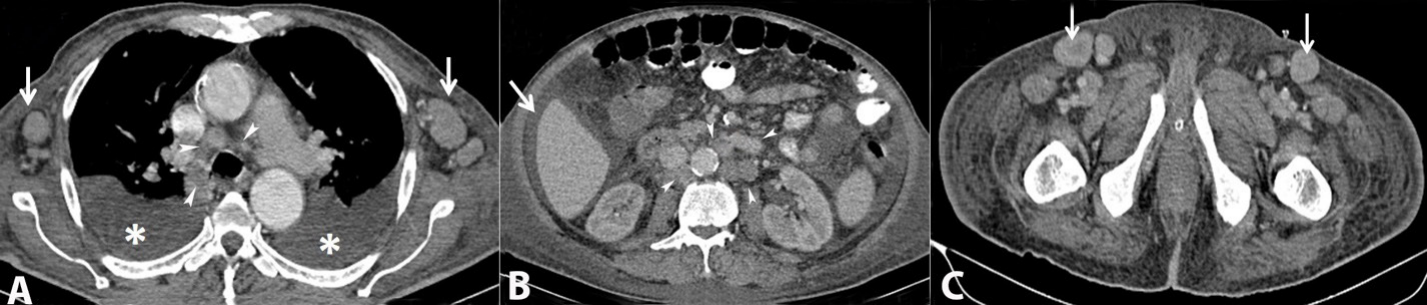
A panel of contrast-enhanced axial CT scan images in soft-tissue window showing: A – Thoracic CT - bilateral pleural effusions (asterisk), bilateral axillary lymphadenopathy (arrows) and mediastinal lymphadenopathy (arrowheads); B – Abdominal CT - ascites (arrow) and retroperitoneal lymphadenopathy (arrowheads); C – Pelvic CT - bilateral inguinal lymphadenopathy (arrows).
